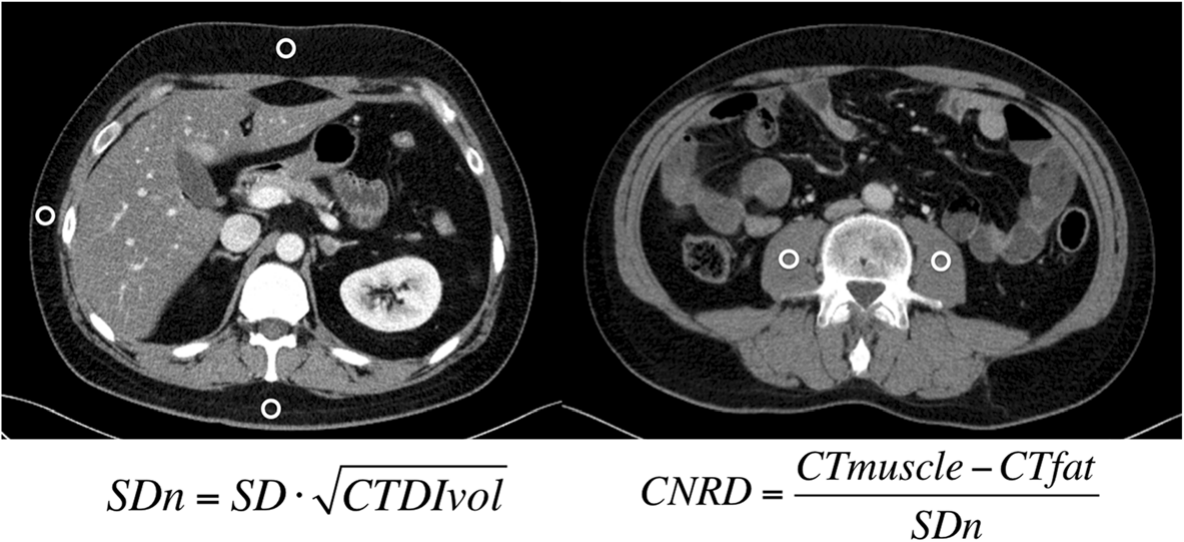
Image noise and contrast-to-noise ratio. *Left:* The image noise (standard deviation (SD) of CT number) was recorded as the mean measurement of three ROIs placed in the subcutaneous fat of anterior, posterior and lateral abdominal wall (CT fat). Image noise was normalized to CTDIvol by $$ SDn=SD\times \sqrt{CDTIvol} $$. *Right:* Mean of CT attenuation of right and left psoas muscle (CTmuscle) was used to calculate the contrast-to-noise ratio (CNRD)
